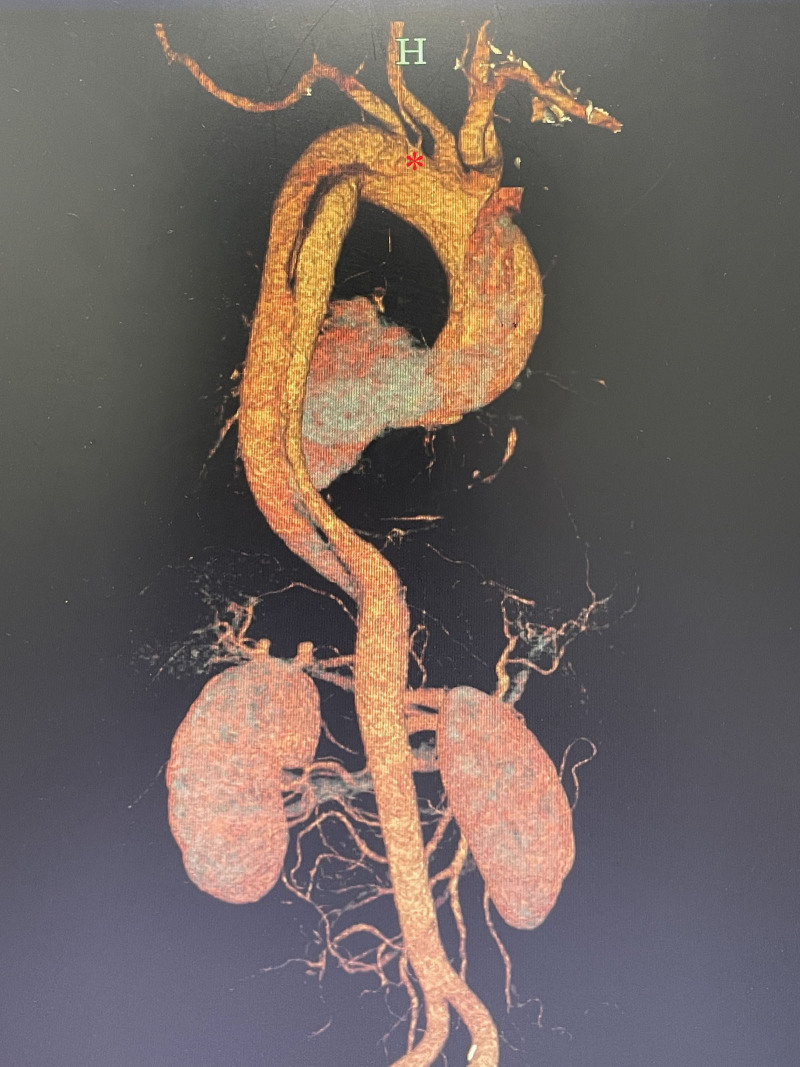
Preoperative CTA indicated that the aortic dissection and the left subclavian artery was derived from the aortic arch. *Isolated left vertebral artery. CTA = computed tomography angiogram.
